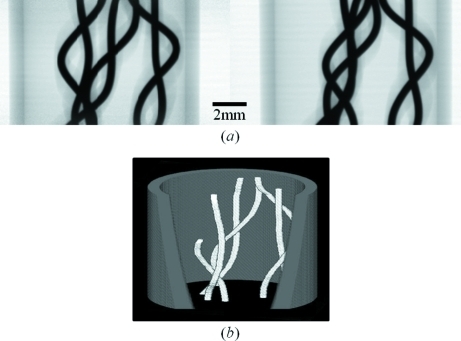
(*a*) X-ray stereo image of six twisted metal wires set in a plastic case. (*b*) Three-dimensional view of the metal wires created from the stereo image in (*a*). The three-dimensional configuration of the plastic case was reconstructed under the assumption that it was a cylinder. It was additionally synthesized into the wire image.
